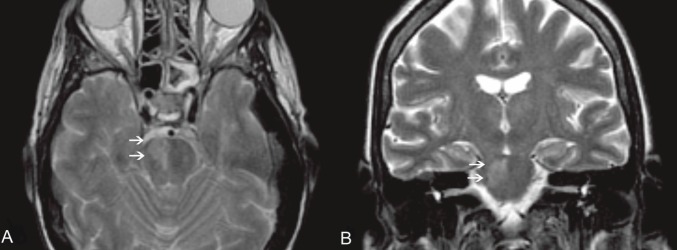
An MRI of the brain, T2 W images, axial (A) and coronal view (B). Clearly visible in the right portion of the pons is a hyperintense area (white arrows), according to pathologic findings (mucormycosis localisation).
